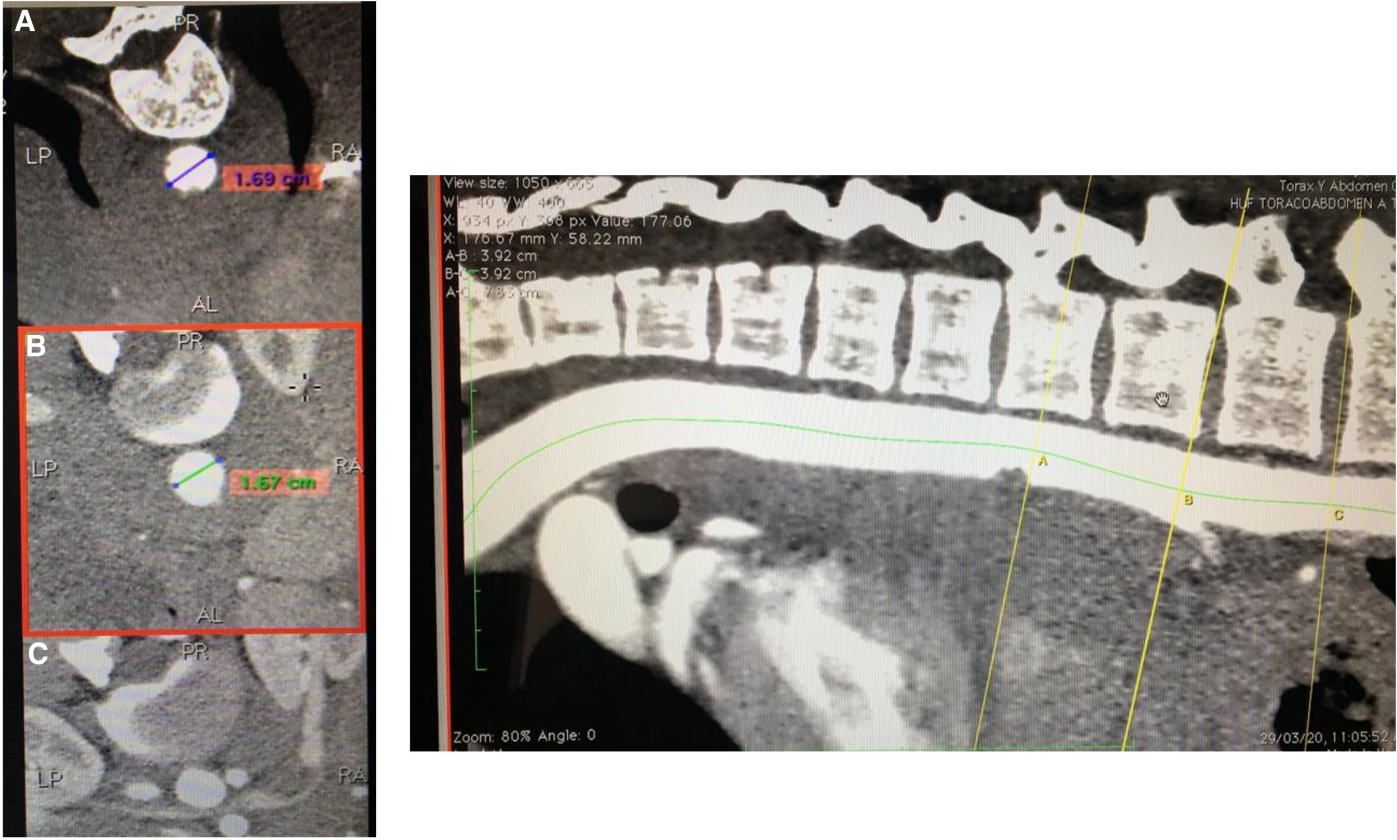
CT angiography measurement of the aorta, distance to the ostium of the celiac trunk.
